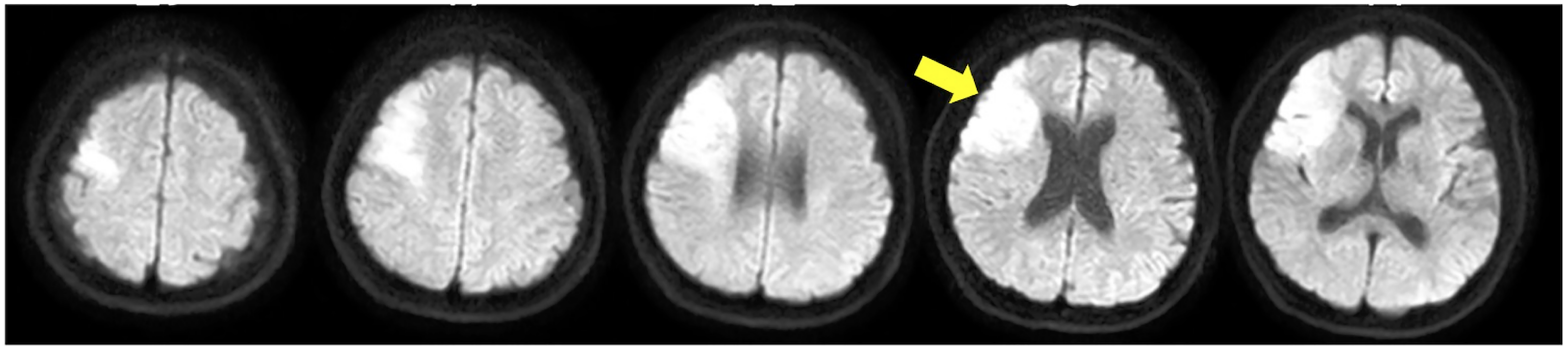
Magnetic resonance imaging scans showing the lesion sites in the patient.
